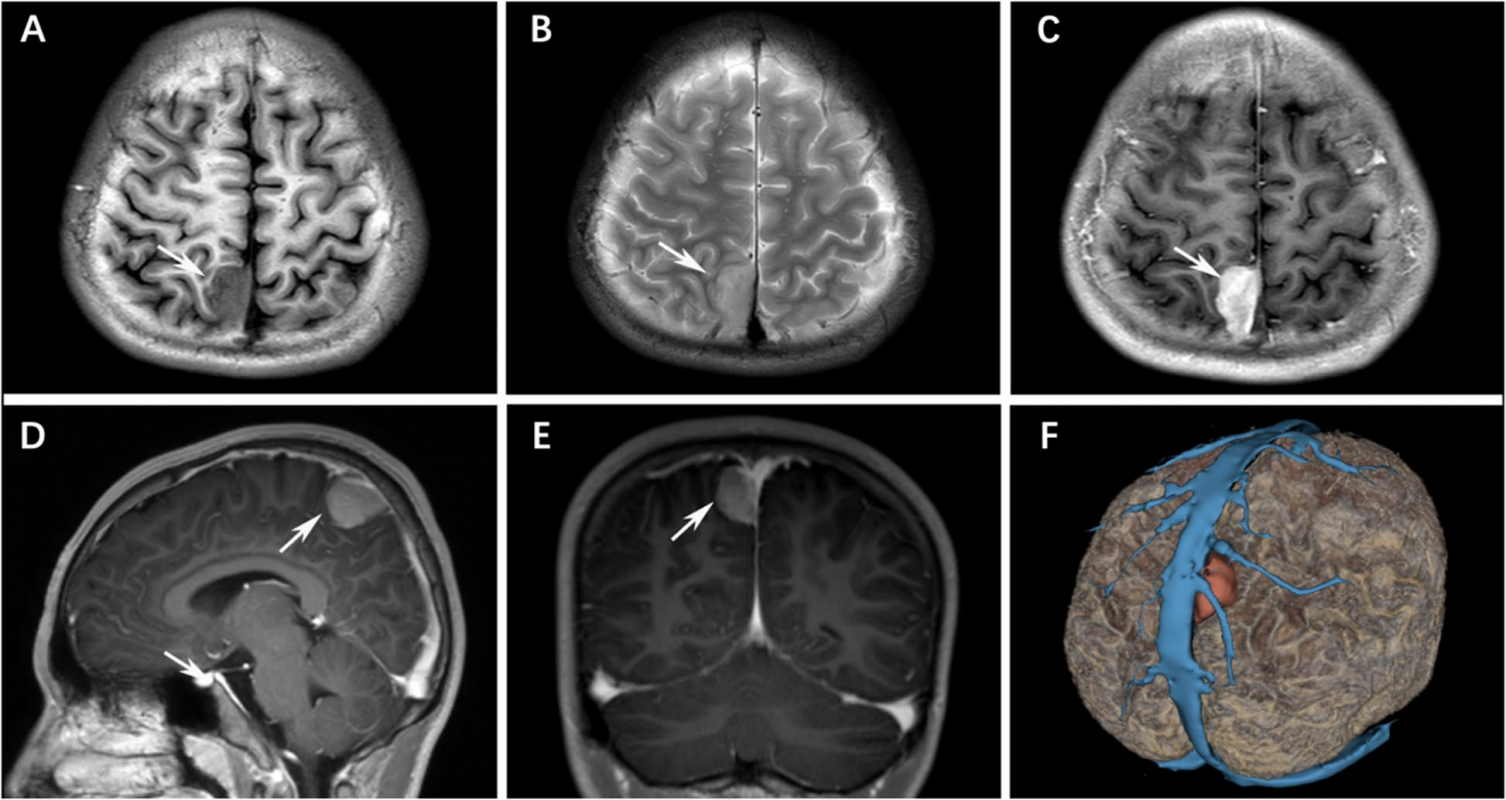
YAP1-MAML2* fusion variant in a 12-year-old boy with meningioma adjacent to the superior sagittal sinus in the right parietal lobe. A-C Axial MRI shows a D-shaped, well-circumscribed and dura-based mass (arrow). The mass demonstrates isointense with cortex on the T1-weighted imaging A hyperintense on the T2WI sequence B and strong enhancement after the administration of Gd-based contrast agent C; D-E Sagittal and coronal T1-weighted MR imaging shows the mass enhances strongly after administration of Gd-based contrast agent, “dural tail” sign could be seen on both sagittal and coronal view. The tumor originates from the lateral wall of the sagittal sinus and the top of the cerebral falx. G Three-dimensional reconstruction is performed using Slicer (http://www.Slicer.org), which showed the upper Trolard vein not invaded by the tumor
